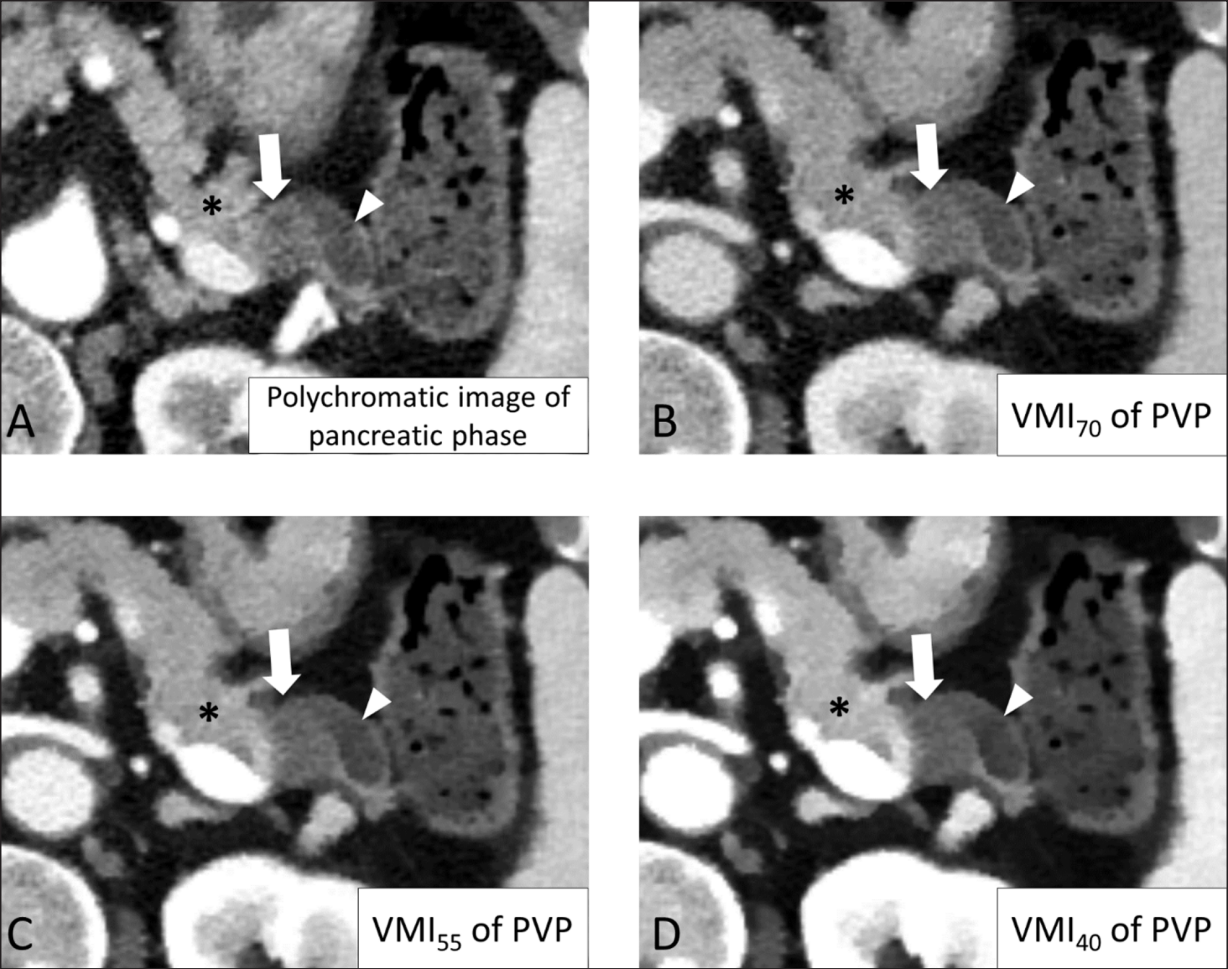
CT images of a 72-year-old woman with pancreatic ductal adenocarcinoma (PDAC). Among the four reconstructed images, VMI_55_ (C) and VMI_40_ (D) showed better contrast between the PDAC (arrow), pancreatic parenchyma (asterisk), and pancreatic duct (arrowhead) than the conventional pancreatic phase (A) and VMI_70_ (B). PVP: portal venous phase; VMI_40, 55, 70_: virtual monoenergetic image at 40, 55, and 70 keV of the PVP.
